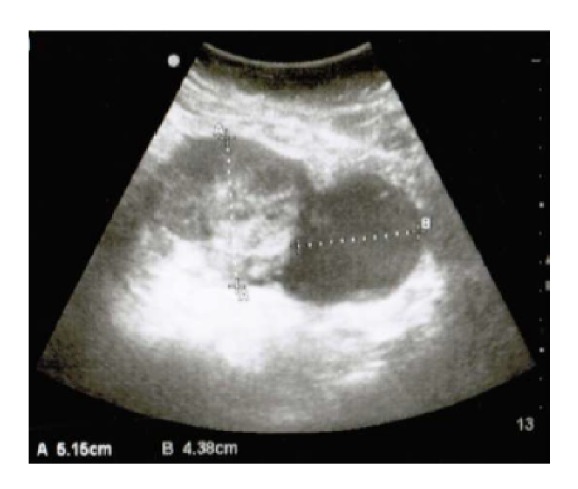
Ultrasonography at 30 weeks showing a complex adnexal mass.
